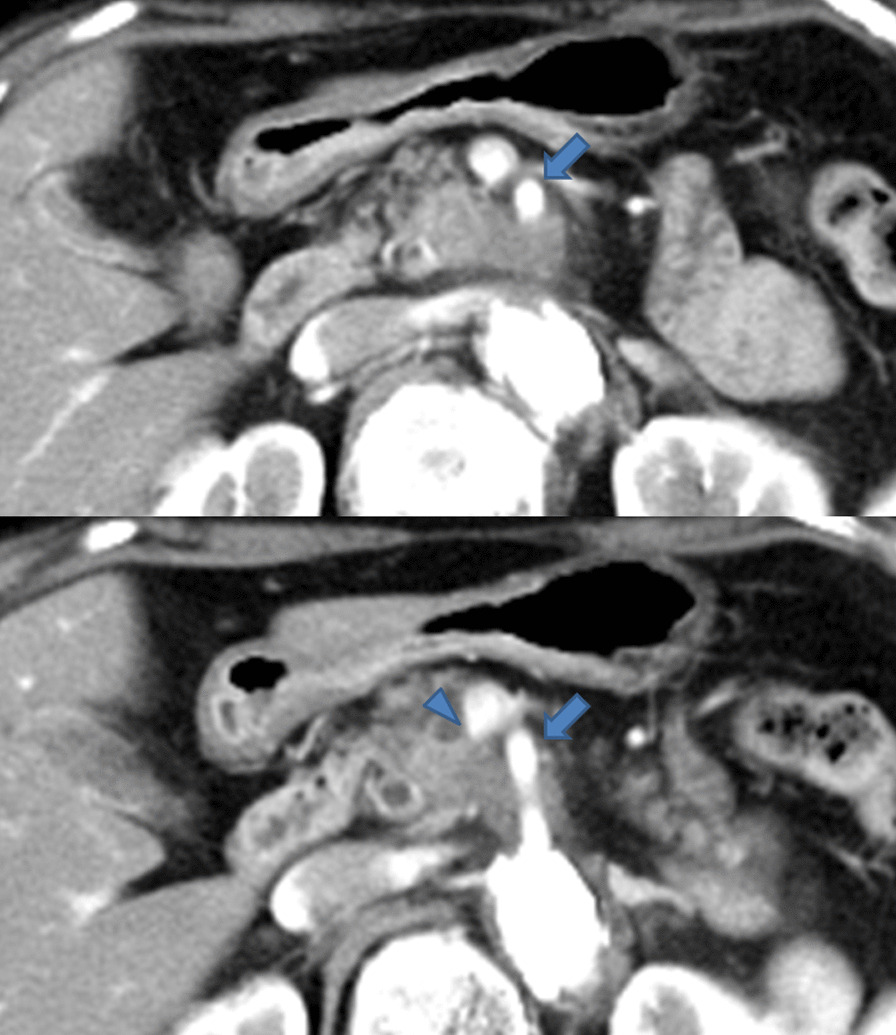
Contrast-enhanced computed tomography findings. CECT revealed a hypovascular tumor in the uncinate process of the pancreas with tumor contact to the superior mesenteric artery (arrow) of more than 180° and portal vein involvement (arrowhead)
